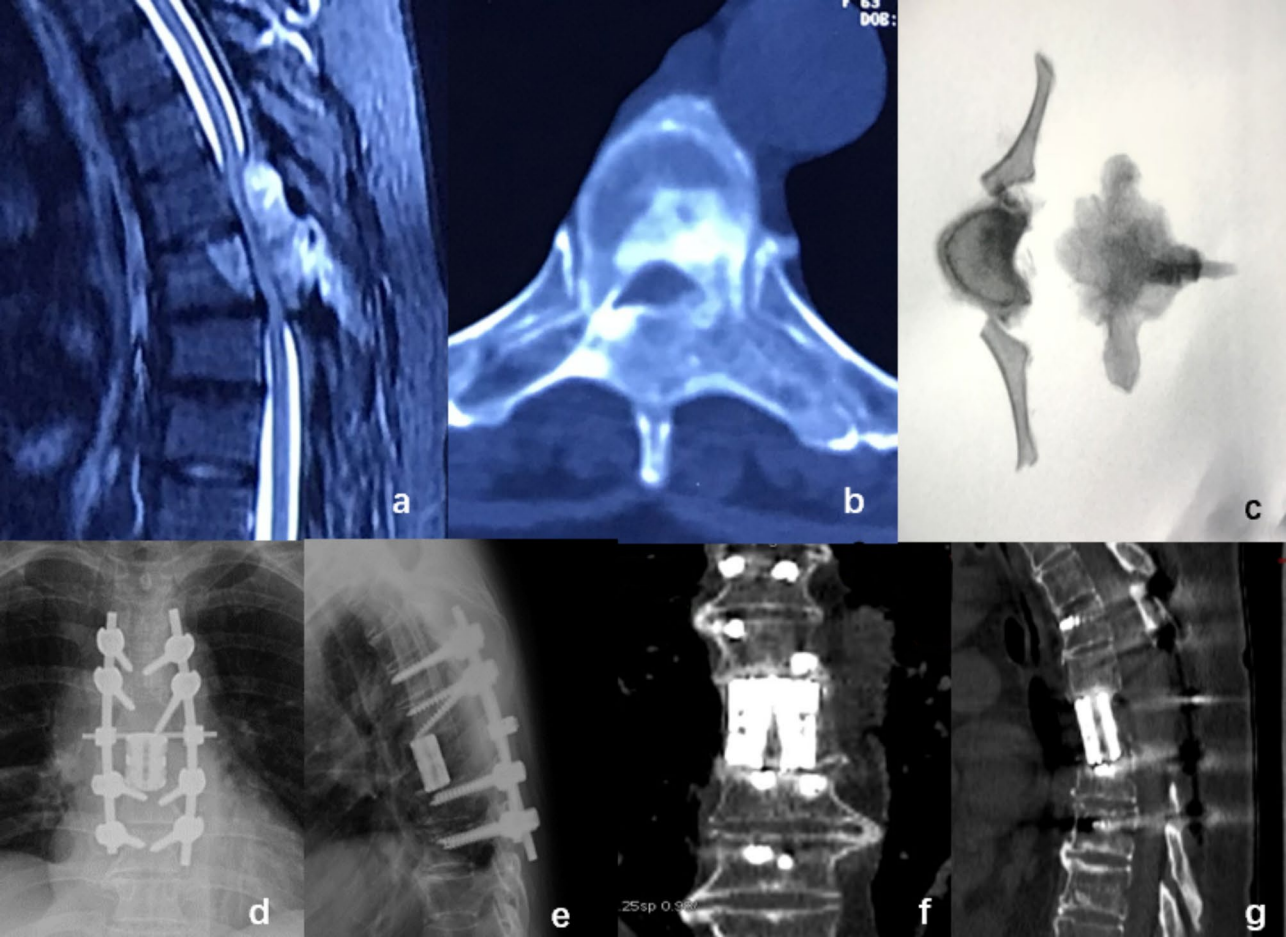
A 63-year-old female patient presented with a thoracic 6 invasive hemangioma. Preoperative CT and MRI scans revealed significant spinal cord compression at the affected segment (a.b). During surgery, the tumor vertebra was completely excised(c). Postoperative X-ray images showed the prefabricated 3D-printed prosthesis was well-positioned (d.e). CT scans at 43 months postoperatively indicated good adherence of the prosthesis to the adjacent endplates, with a subsidence of 2 mm, no screw loosening, and no internal fixation breakage(f.g)
